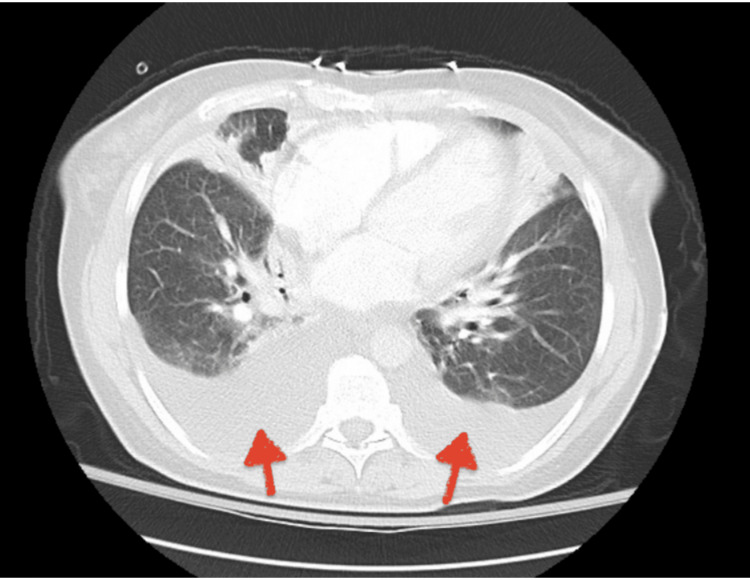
Computed tomography angiography (CTA): Axial view showing significant bilateral pleural effusion (red arrows).
